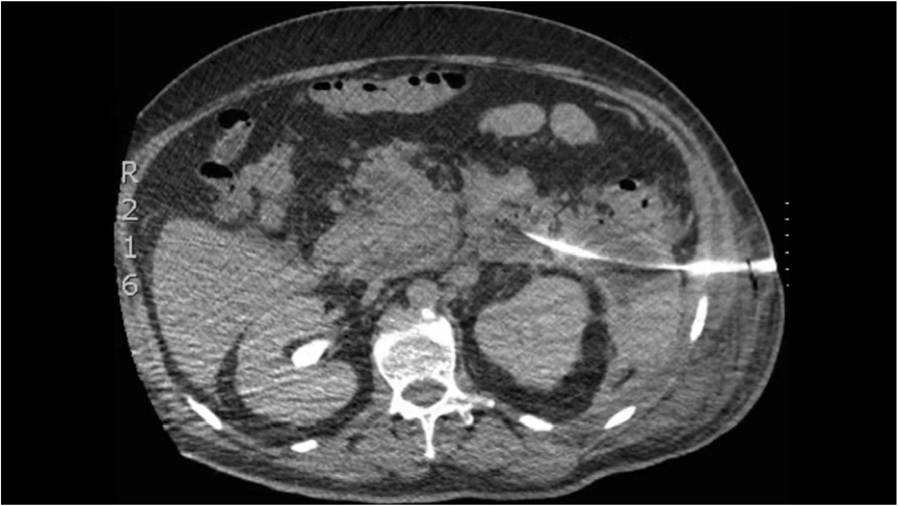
CT scan previous percutaneous SEMS placement
